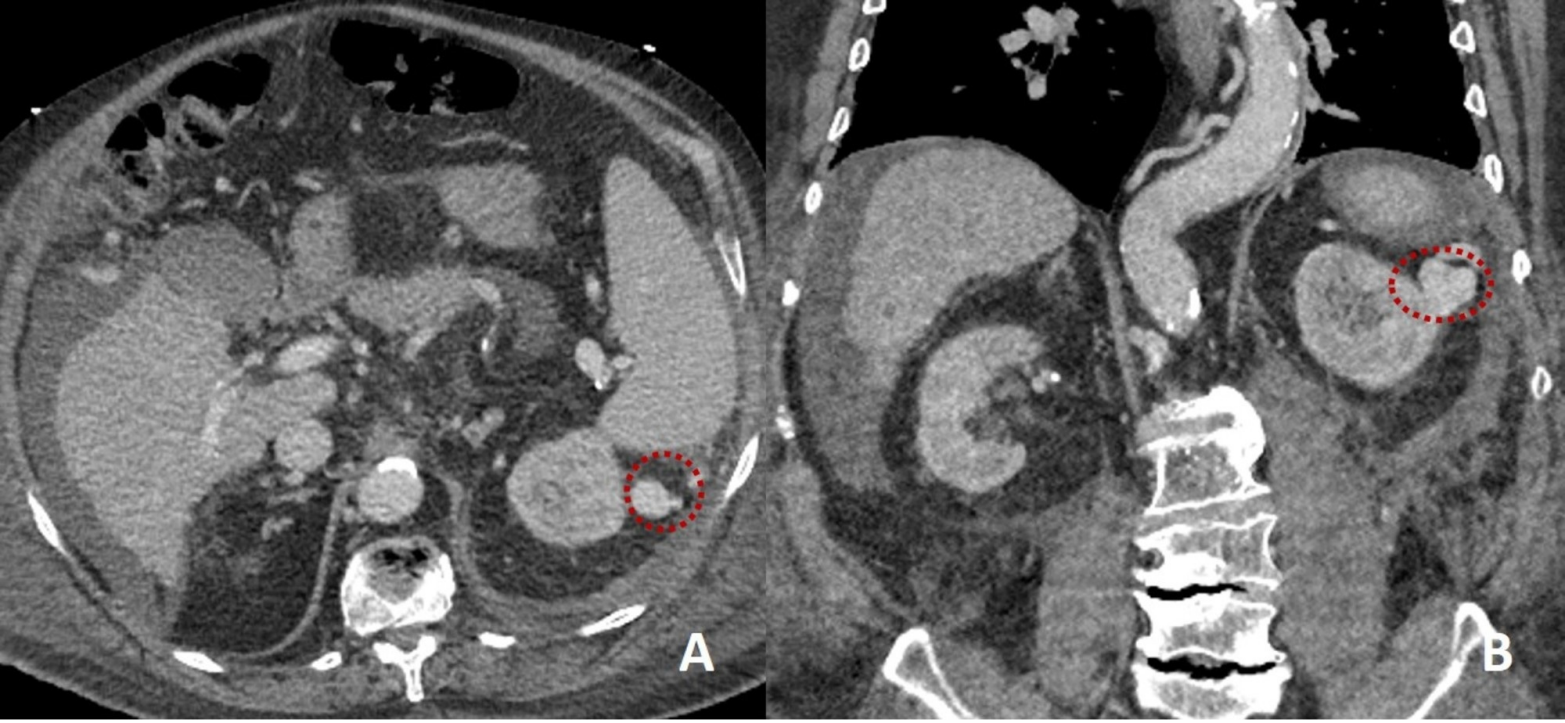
Computed tomography of the abdomen showing a very tortuous splenorenal shunt, which coursed about the spleen laterally and possessed a notable dilated varix maximally measuring 22 mm. Panel A showing axial view and Panel B showing coronal view
